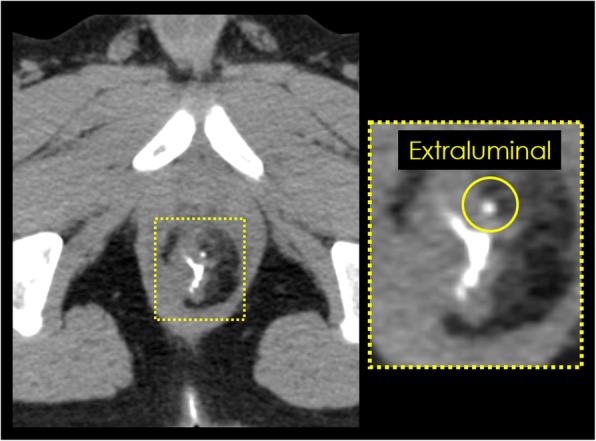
Pelvic plain computed tomography (CT) without contrast medium. Retrograde infusion of Gastrografin® was detected extraluminally on CT image, and this case was diagnosed as an occult anastomotic leakage
